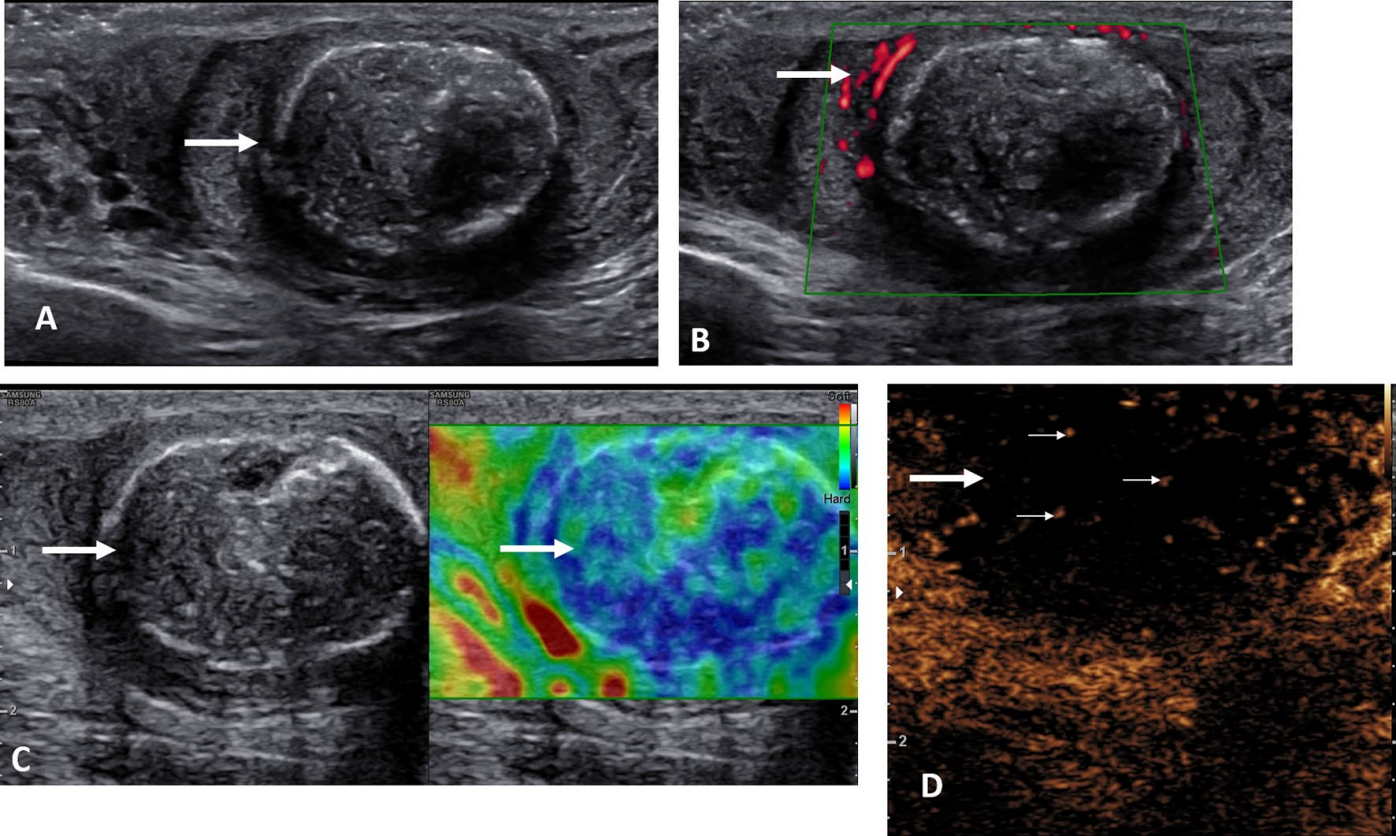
A 46-year-old with a right testicular lump, with a epidermoid cyst. A A longitudinal image of the right testis, with a heterogenous (arrow) occupying the central aspect of the testis, with an echogenic border, and areas of calcification. B The microvascular image demonstrating color flow (arrow) around the heterogenous lesion but no internal flow. C The tissue elastography image shows that the lesion is stiffer than the surrounding normal tissue (arrows). D A contrast enhanced ultrasound examination shows an avascular epidermoid cyst (large arrow) with artifact (small arrows) of high reflectivity from echogenic areas; there is no vascular flow present
